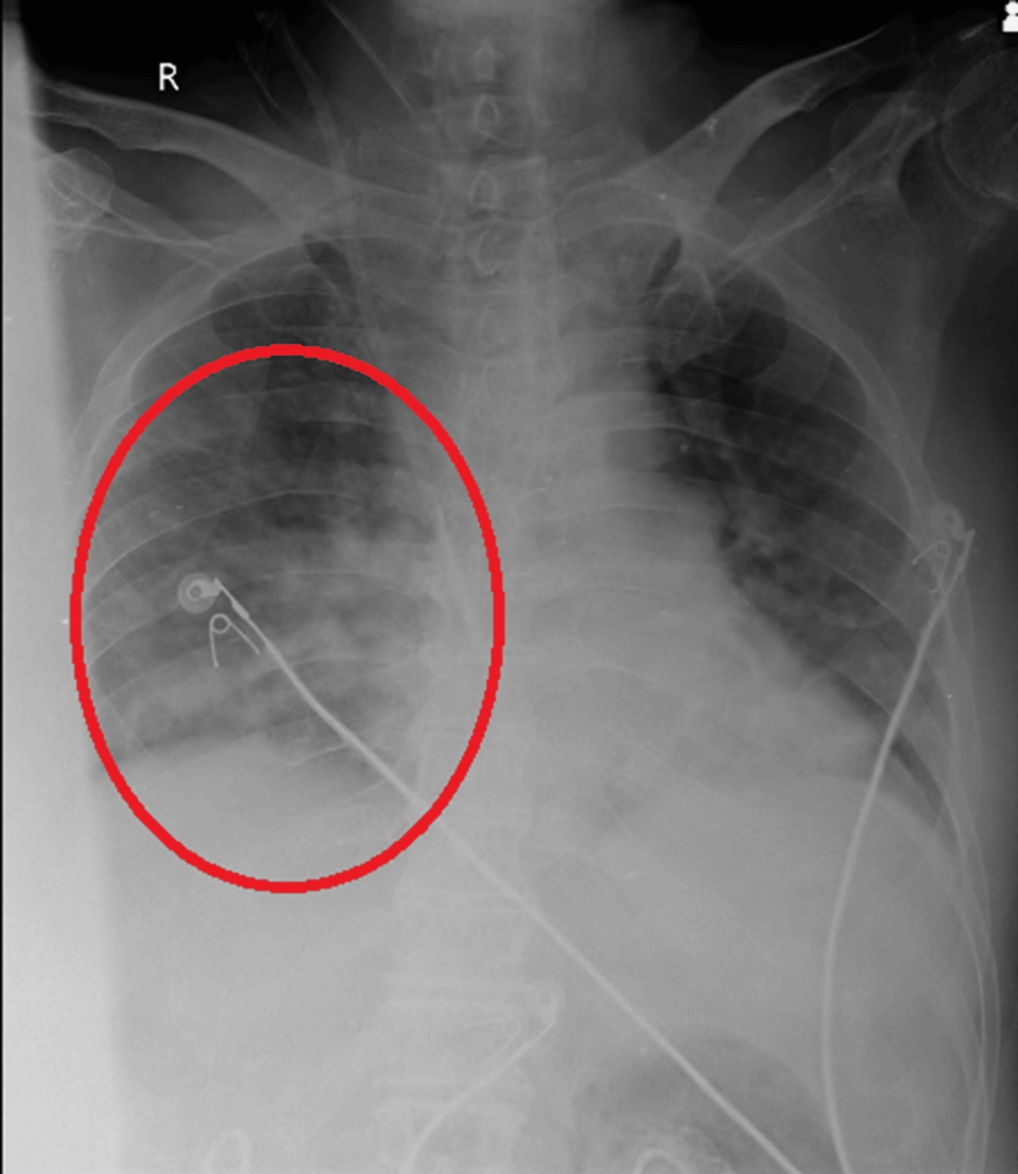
Post-physiotherapy rehabilitation X-ray.
